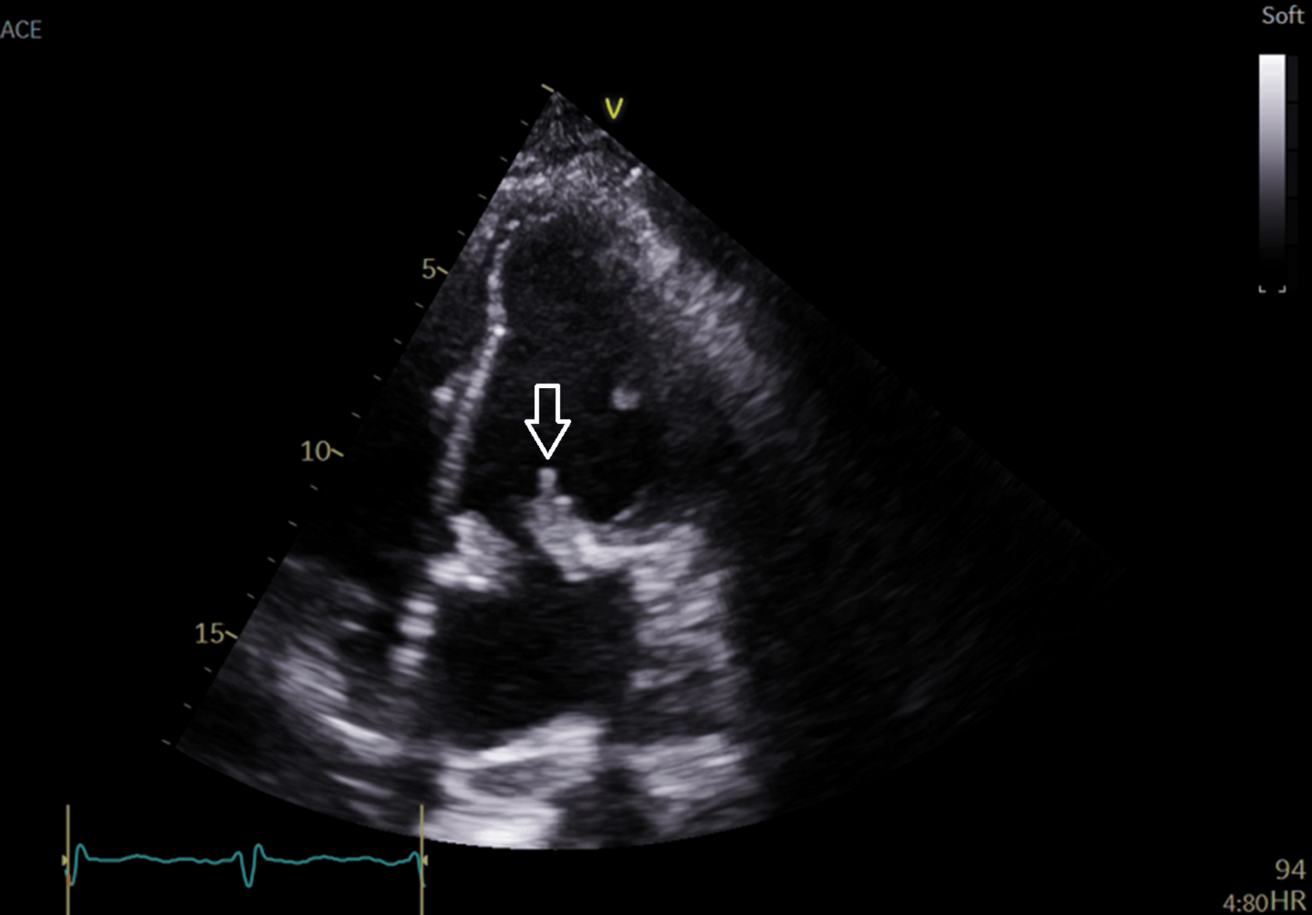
Shows AP4C view with prosthetic valve vegetations (white arrow) AP4C: apical four-chamber
